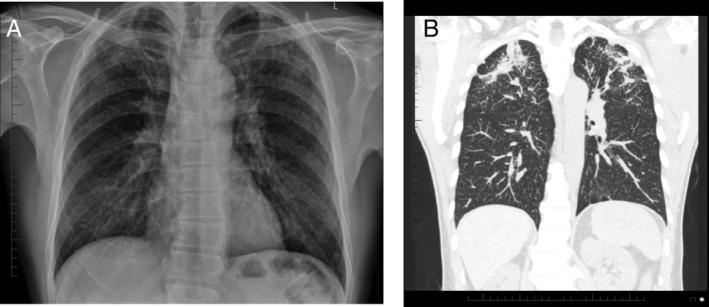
(A) A chest X‐ray reported with the International Labour Organization (ILO) standards in a tunneller with 30 years of dust exposure demonstrates silica nodules >3 mm in diameter with an ILO grade of 2/3. (B) Bilateral progressive massive fibrosis in the same tunneller was confirmed on a subsequent non‐contrast computerized tomography (CT) chest. This CT shows bilateral fibrotic masses with associated architectural distortion and volume loss on a background of diffuse solid silica nodules.
